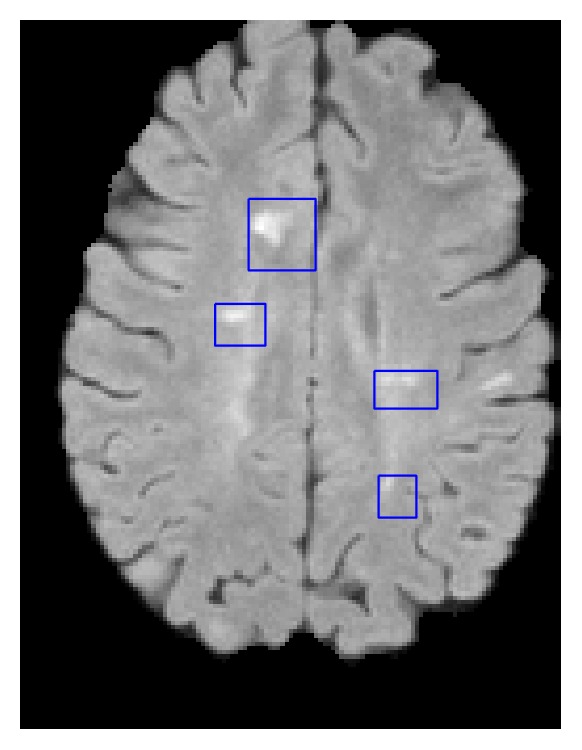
ROI (blue) on a FLAIR image.
